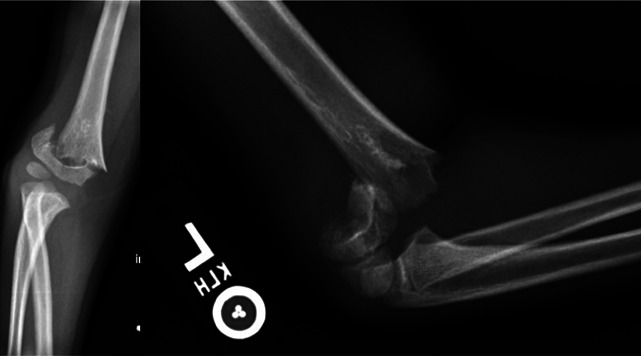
Anteroposterior and lateral radiographs of a Gartland Type III fracture with posterolateral displacement and rotation is demonstrated. Supination and traction will improve axial and coronal alignment, respectively, before flexing the fracture to restore the sagittal plane.
